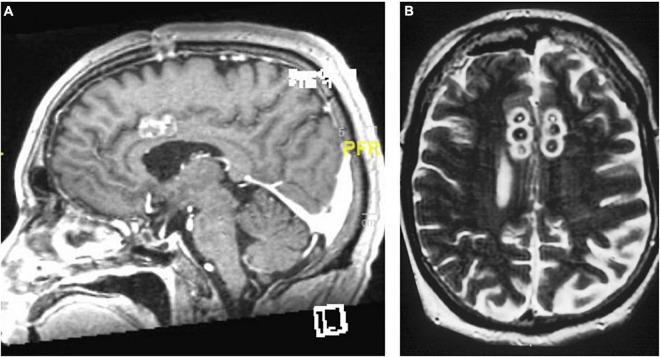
The “six-pack” cingulotomy. (A) Sagittal and (B) Axial T2-weighted MRI of chronic triple lesion cingulotomy.
